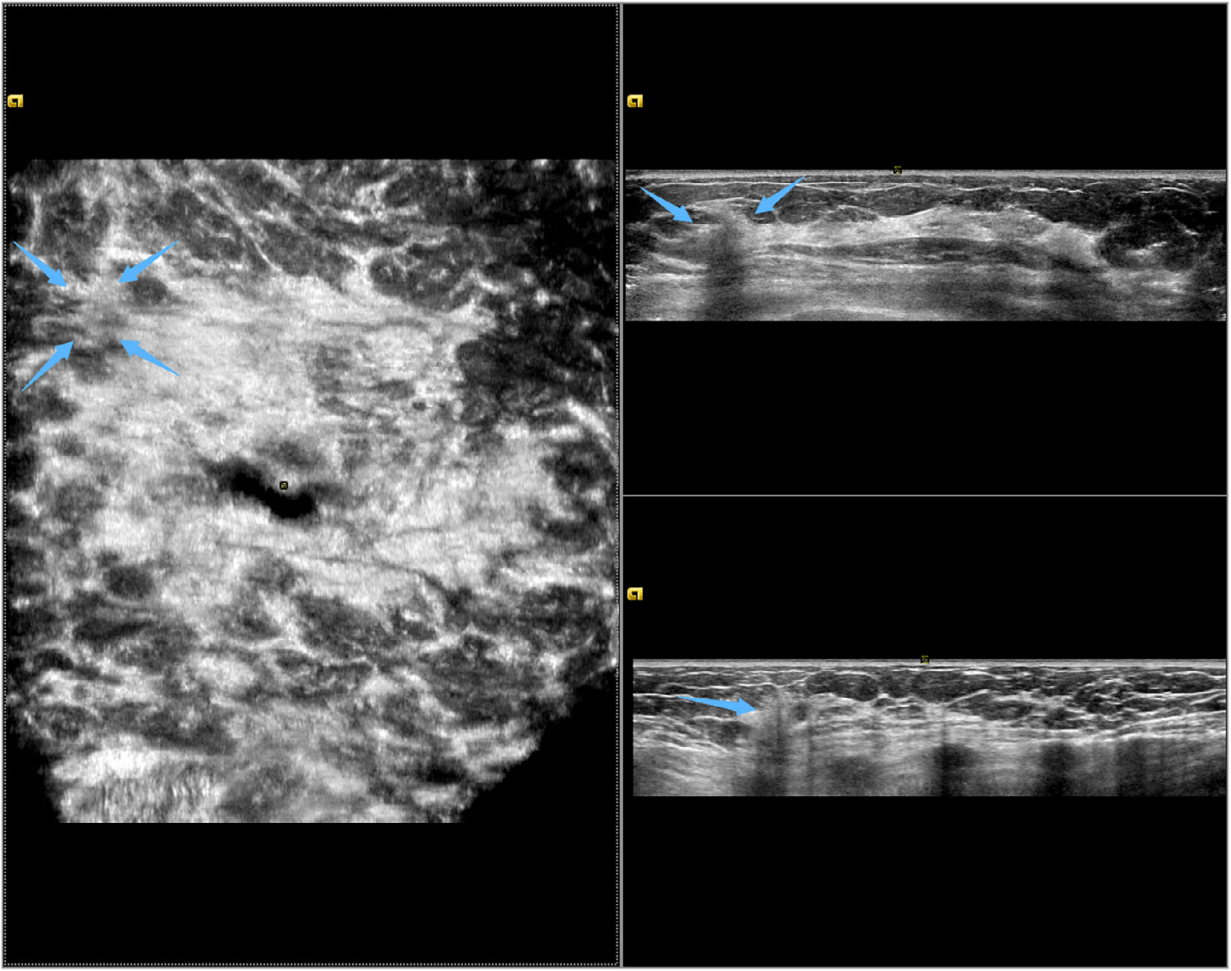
The left breast lesion in automated breast ultrasound (US). The left anteroposterior view reveals a non‐mass‐like breast lesions in the 10‐ to 11‐o'clock position 7 cm away from the nipple (blue arrows)
